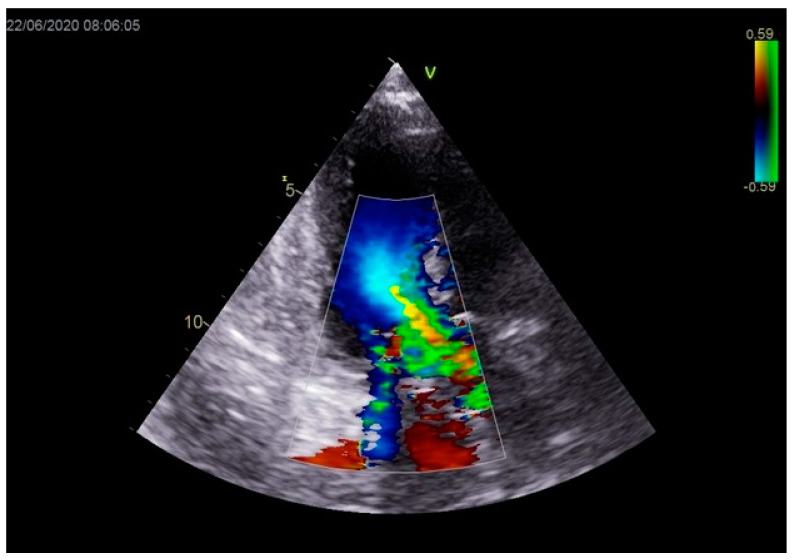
Mild mitral regurgitation was registered with color Doppler echocardiography.
